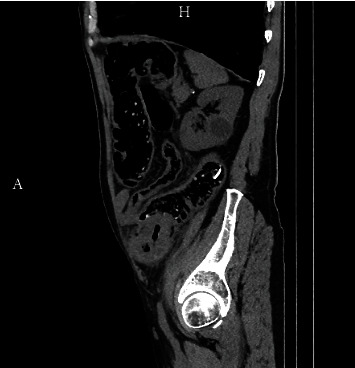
The tumor in the sigmoid fixated to the abdominal wall seen on CT in lateral cross-section.
